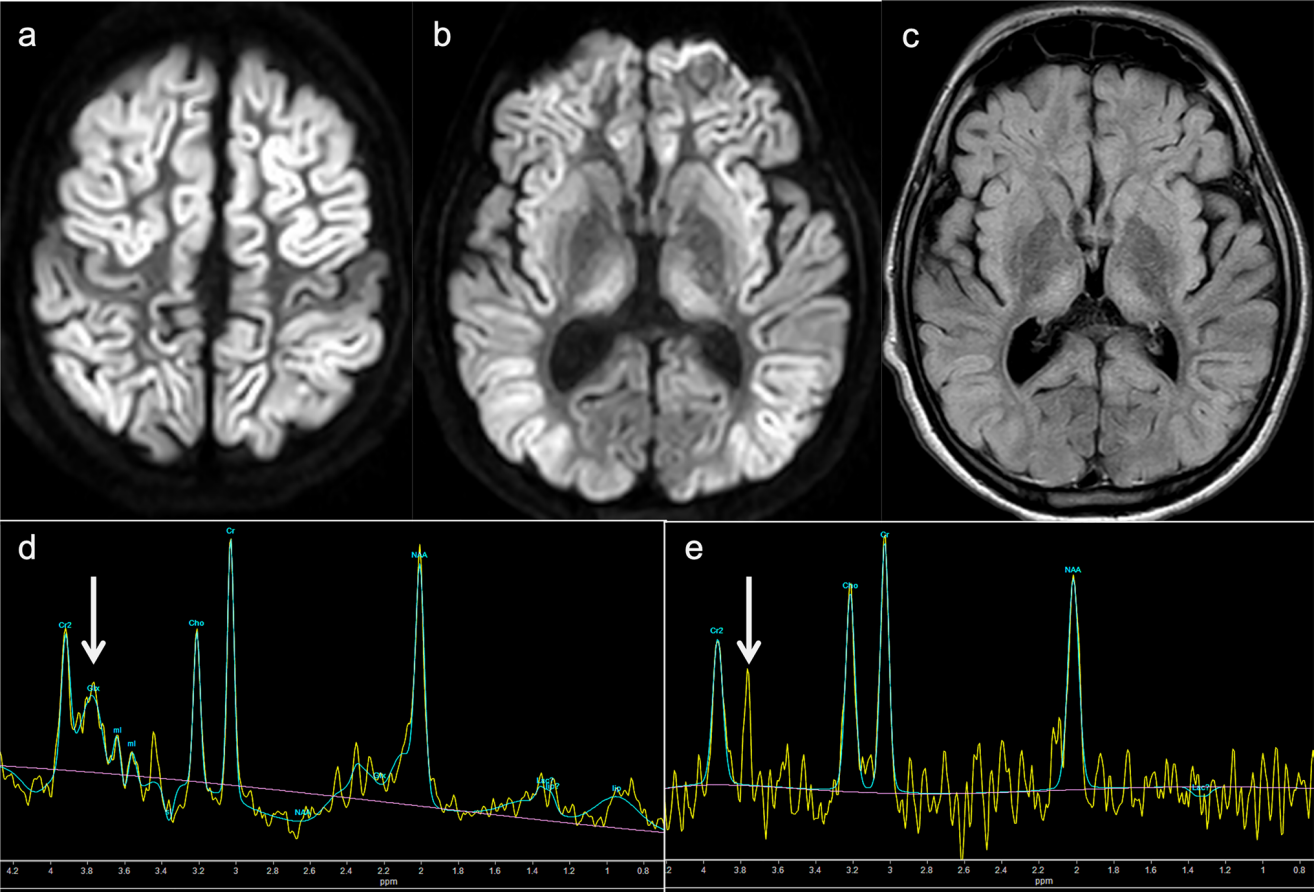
Arginase (ARG1) deficiency. A 16-year-old, male patient with developmental delay and epilepsy. Five days after receiving the COVID-19 vaccine, acute hyperammonemic encephalopathy developed. a-c DWI (a, b) and FLAIR images (c) demonstrated diffuse, high-signal areas in the bilateral cortices (the peri-central sulci are unaffected). Symmetrical, high-signal areas were also seen in the bilateral striata and medial thalami. Diffuse brain atrophy was also observed. d, e ^1^H-MRS (TE = 35, 144 ms) of the affected right thalamus displayed elevated arginine at 3.8 ppm, which was clearly visible at 144 ms (e) (arrows)
